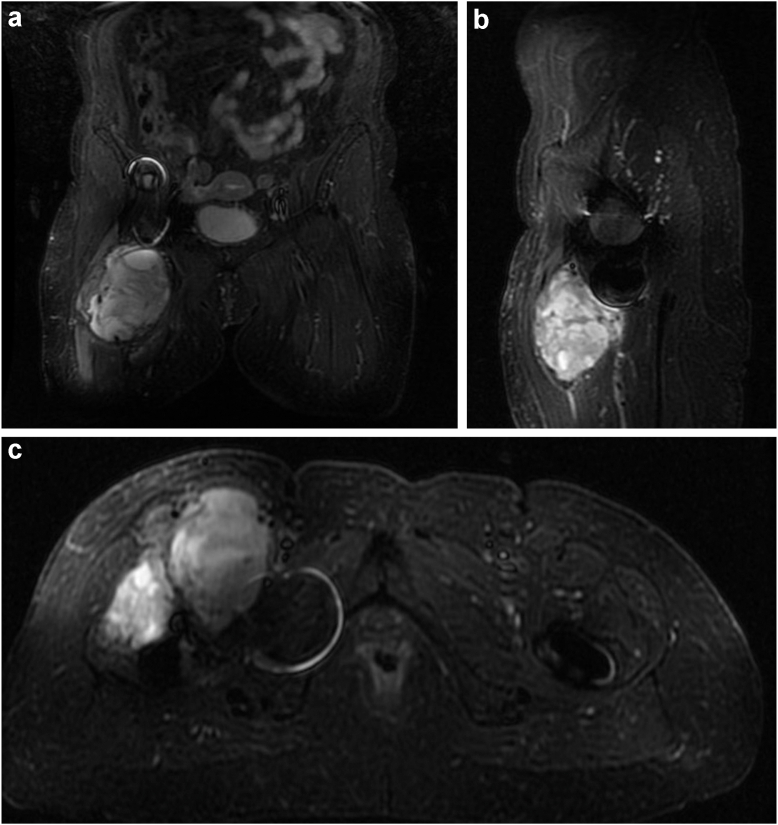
(a) Coronal, (b) sagittal, and (c) axial MRI images demonstrating a lobular, irregular, large mass anterior to the proximal femoral shaft with high signal intensity on fluid-weighted sequencing.
